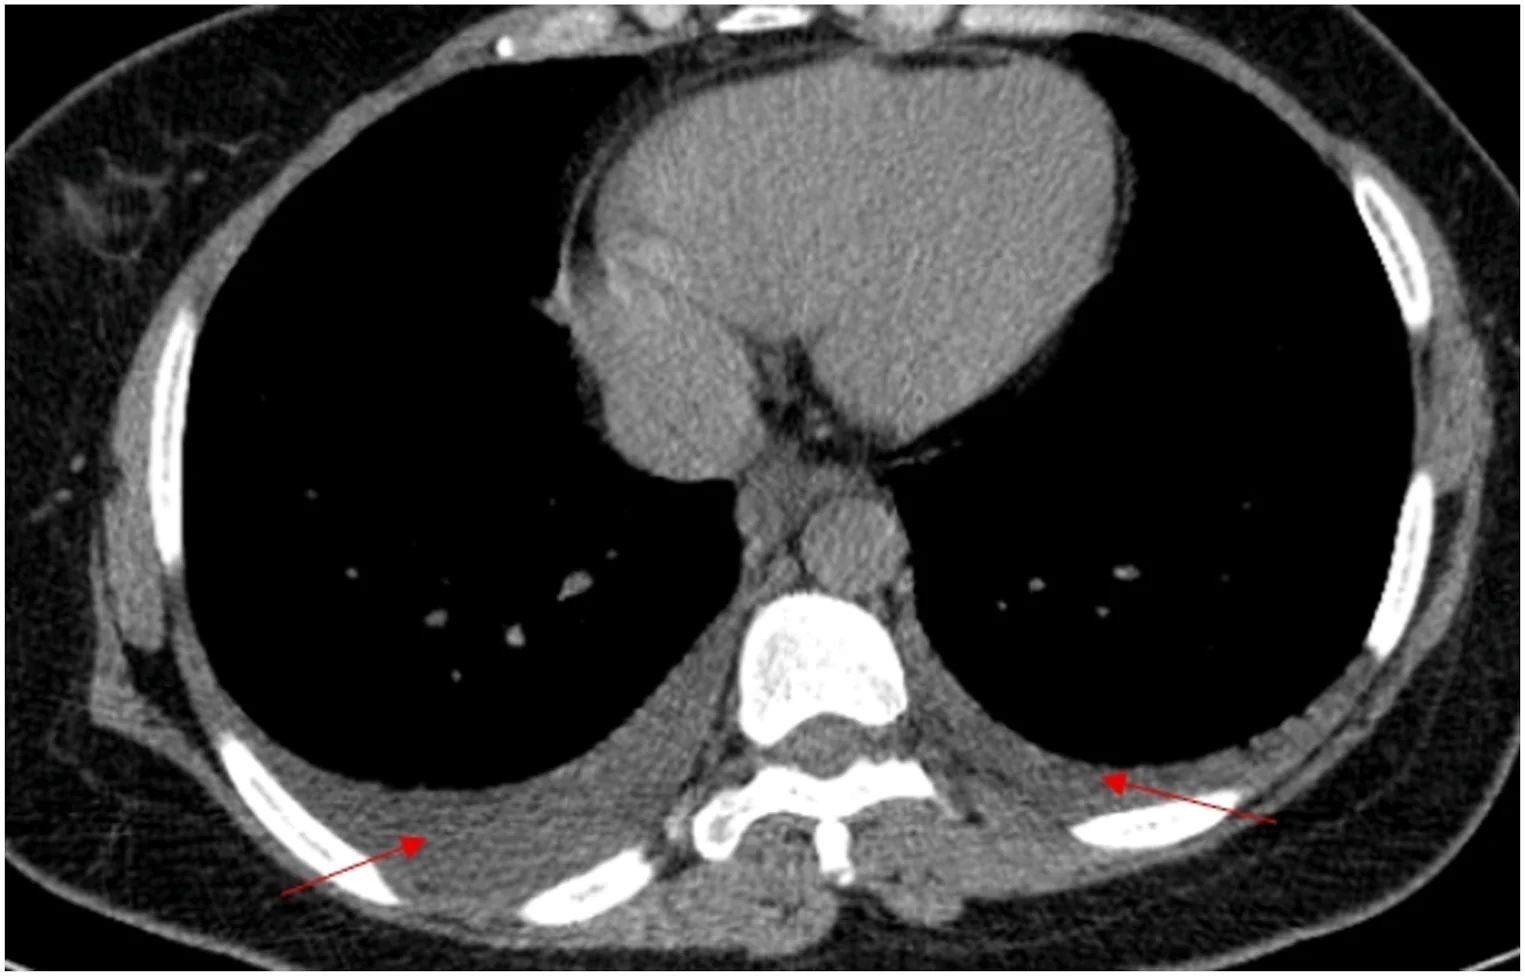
Chest CT soft tissue window axial plane shows bilateral pleural effusion (arrows), with a massive collection on the right side, likely representing chylous effusion.
